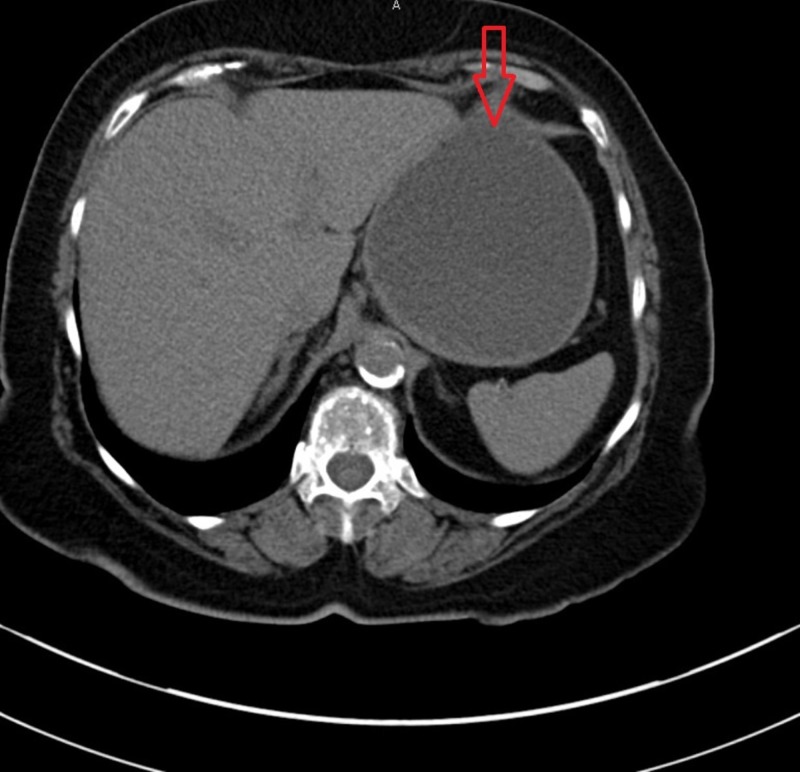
A computed tomography (CT) scan of the abdomen and pelvis showing a dilated stomach with gastric outlet obstruction.
